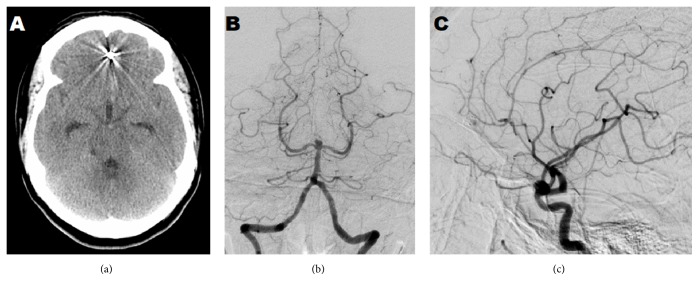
Imaging upon hospitalization in 2016. (a) CT head without contrast in 2016 showing intracerebral hemorrhage adjacent to the previously coiled aneurysm. (b and c) Cerebral angiogram of the anterior and posterior circulations showing the development of multiple new aneurysms.
